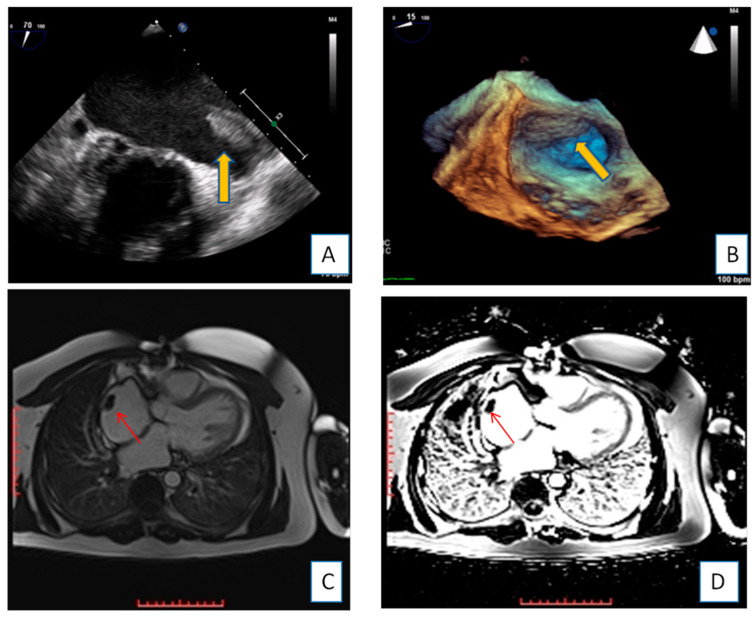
Transesophageal echocardiography depicting the left atrial appendage using 2D and 3D approach ((A,B), respectively). A huge thrombus (arrows) is demonstrated in the upper part of the “coumadin ridge” is an elderly woman with severe mitral stenosis. Clot in the RA (red arrows). Cine and EGE imaging ((C,D) respectively).
